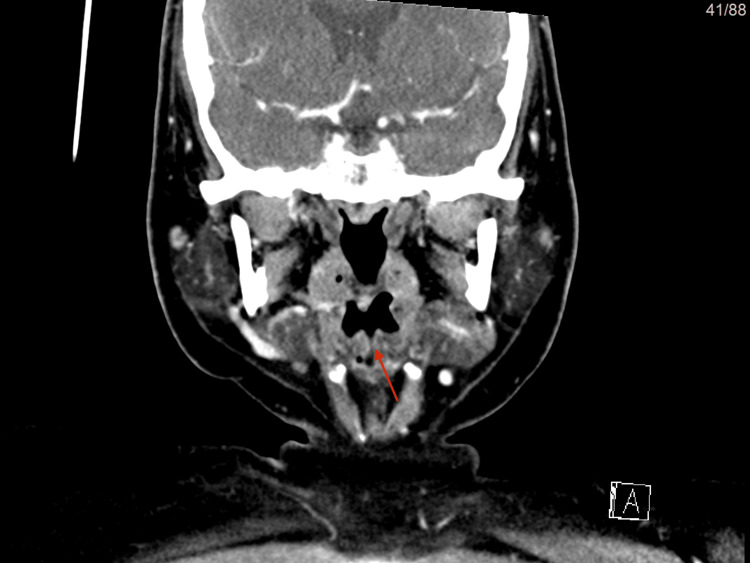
(CT scan) in this coronal view The red arrow shows the bifid epiglottis in a more posterior view.
